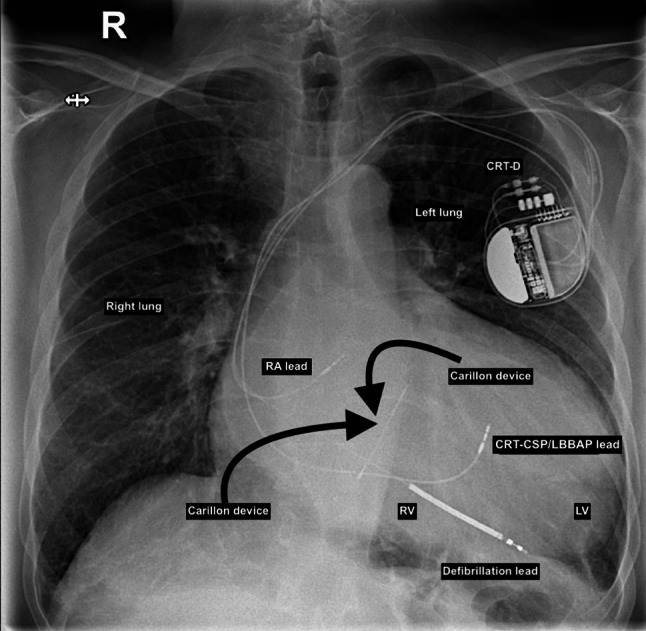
Chest X-ray cardiomegaly with CRT-CSP/LBBAP and Carillon device implantation in coronary sinus. *RA *Right atrium, *RV *Right ventricle, *LV *Left ventricle, *CRT *Cardiac resynchronization therapy, *CSP *Conduction system pacing, *LBBAP *Left bundle branch area pacing
